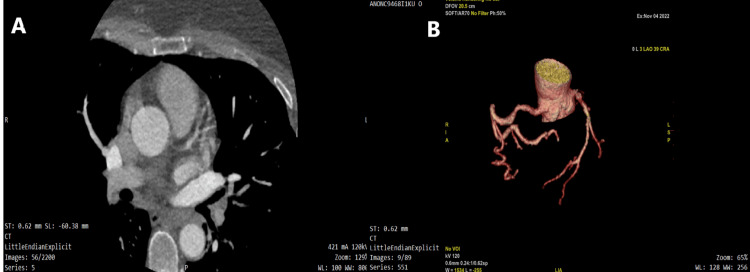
A and B: Multidetector coronary computed tomography images.
